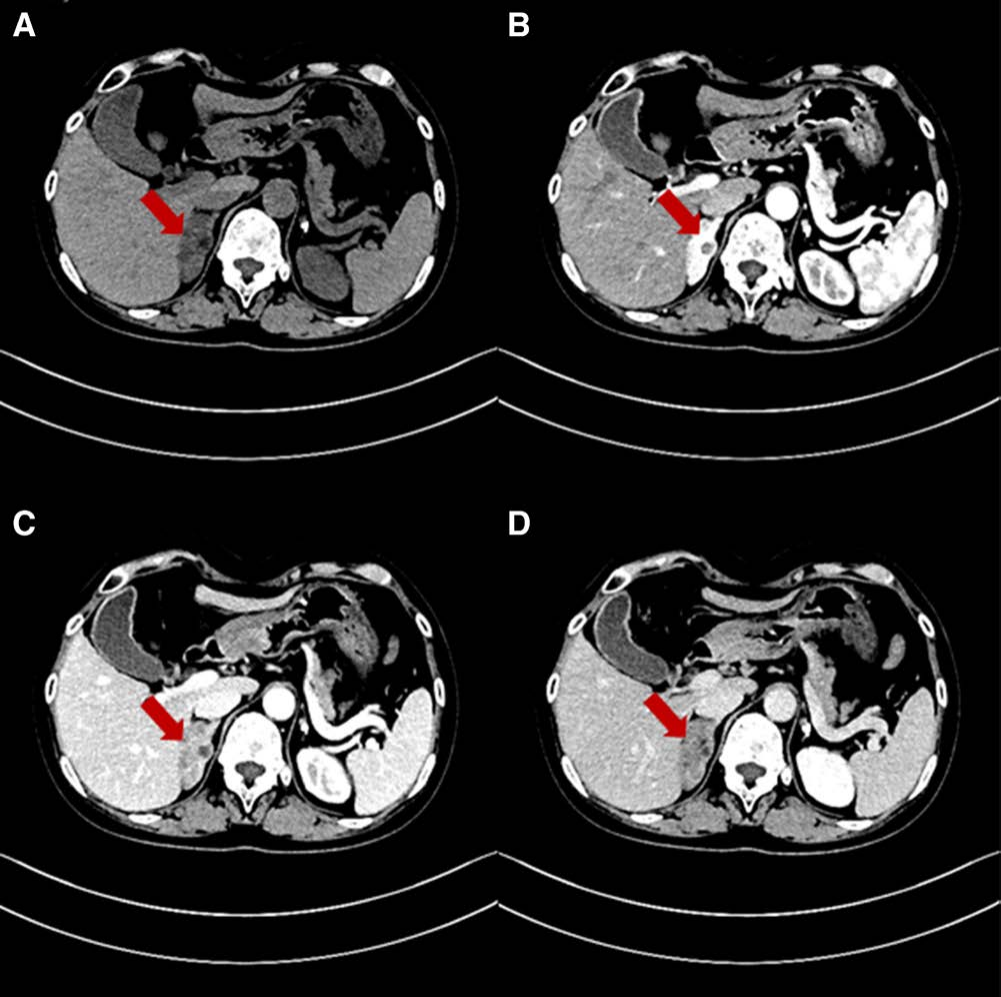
Adrenal enhanced CT results. (A) Normal sweep phase. (B) Arterial phase. (C) Venous phase. (D) Excretory phase. The red arrow indicates the enlarged adrenal gland. CT = computed tomography.
